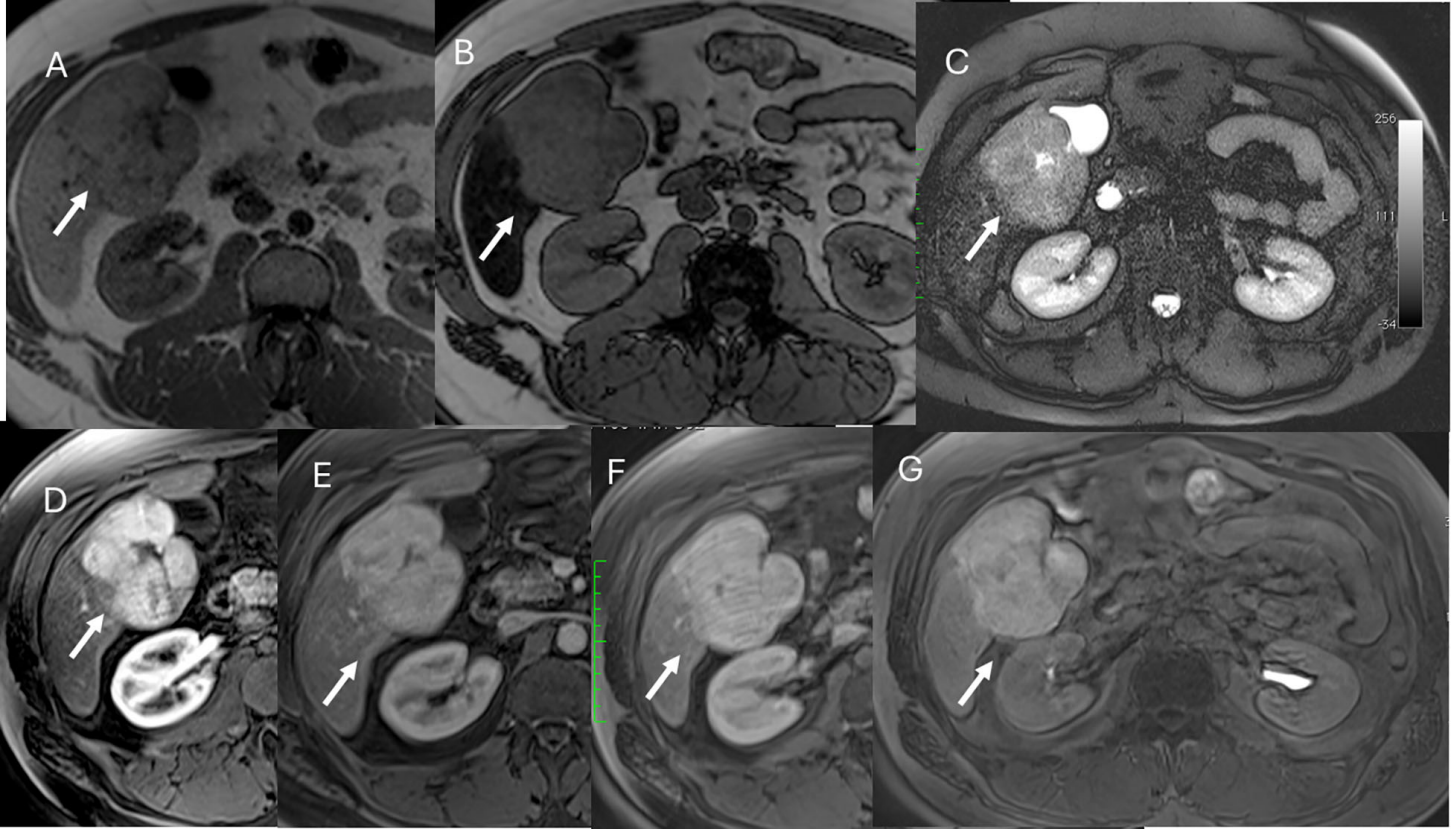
MRI assessment of FNH lesion (arrows) with Gd-EOB-DTPA contrast. In (A) (in-phase) and (B) (out-phase), the lesion shows hypointense signal with central scar and steatosis of the liver parenchyma in (B). In C (T2-W FS sequence), the lesion is hyperintense with central scar. During contrast study, the lesion shows non-rim hyperenhancement during arterial phase (D), no washout during portal (E) or transitional (F) phases, and hyperintense signal on hepatospecific phase (G).
